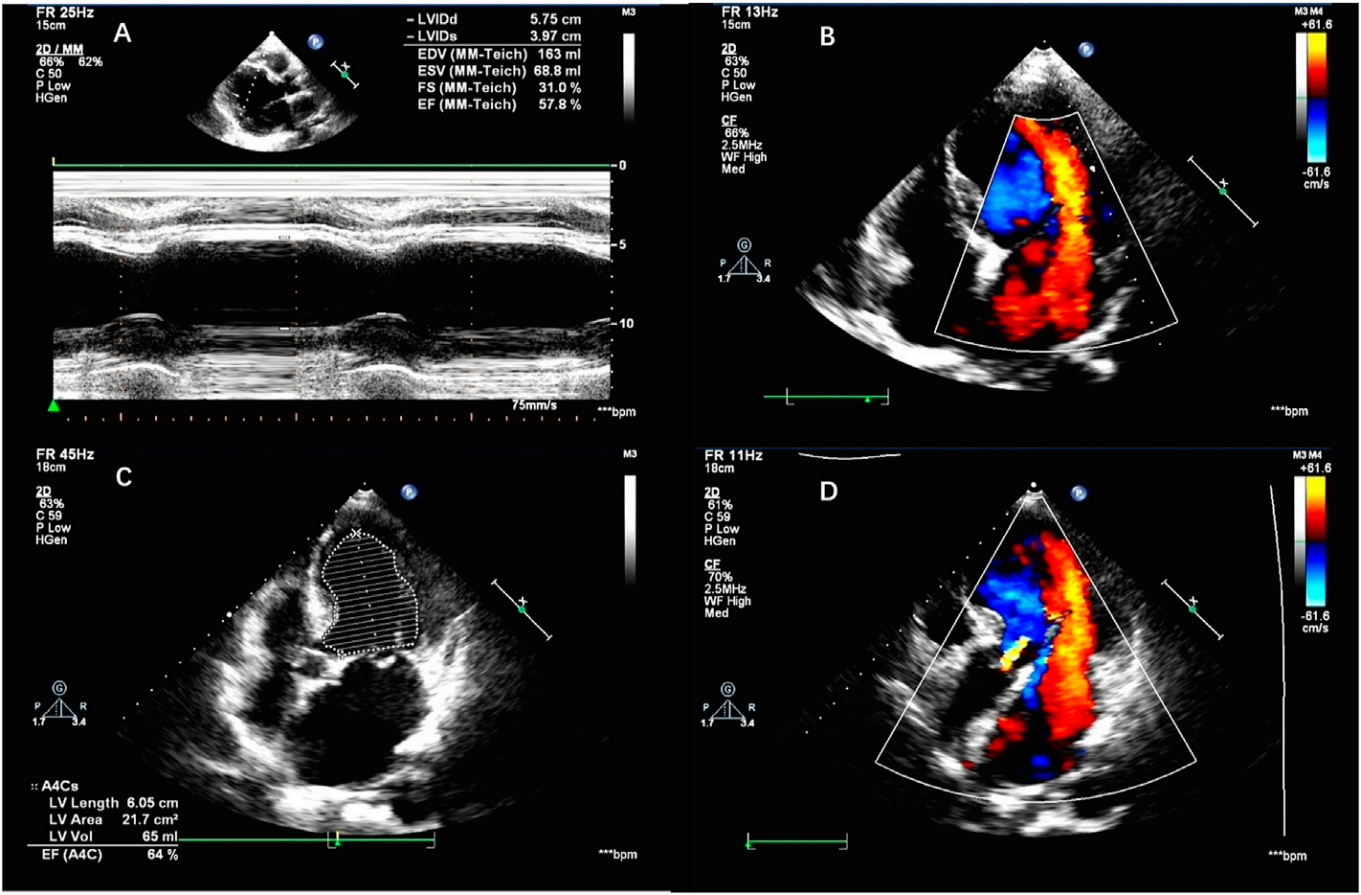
(A,B) Echocardiography before the antitumor therapy. (C,D) Echocardiography after the five-course antitumor therapy.
